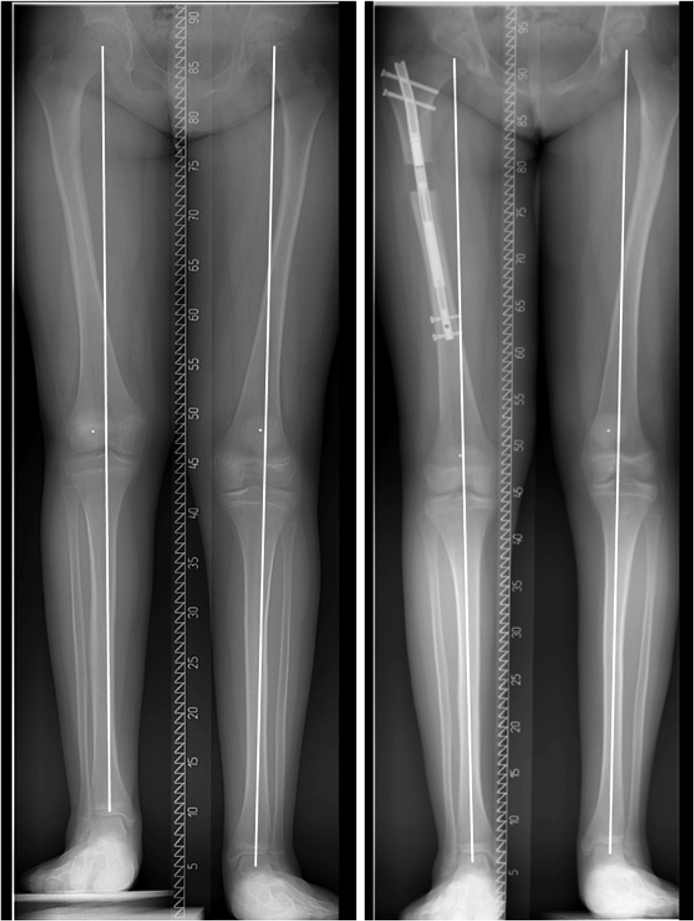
11-year-old girl with cerebral paresis and hemiplegia on her left side. Initial leg length discrepancy was 3 cm, where the hemiplegic side was the longer extremity. She had serious gait problems and gait analysis with shoe augmentation of 4 cm on the right side showed significant improvement of gait parameters. The girl was lengthened 4 cm in her right femur, overcorrecting her by 1 cm, which was advantageous with respect to her left-sided hemiplegia and future growth. Long standing radiographs demonstrate that no shift of MA axis was observed.
